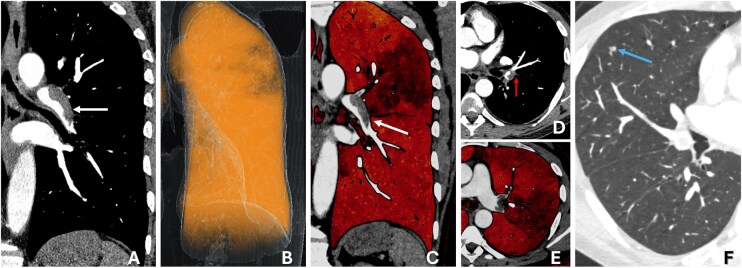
A 46-year-old male underwent a high-pitch multienergy pulmonary embolism (PE) study with PCD-CT showing acute bilateral pulmonary emboli, including a left lobar embolus (white arrows in A and C) causing complete occlusion of the superior lingular artery (red arrow in D). The overall embolic burden is demonstrated on the PCD-CT perfusion maps (reduced yellow color in B and focal dark-red perfusion defects in C and E), with multiple areas of hypoperfusion corresponding to vascular territories distal to the embolism. High-pitch acquisition reduces motion artifacts, permitting imaging of the chest in a single second, with a 0.6-mm slice thickness demonstrating a small 3-mm solid pulmonary nodule in the right middle lobe (blue arrow in F). Abbreviations: PCD, photon-counting detector; PE, pulmonary embolism.
